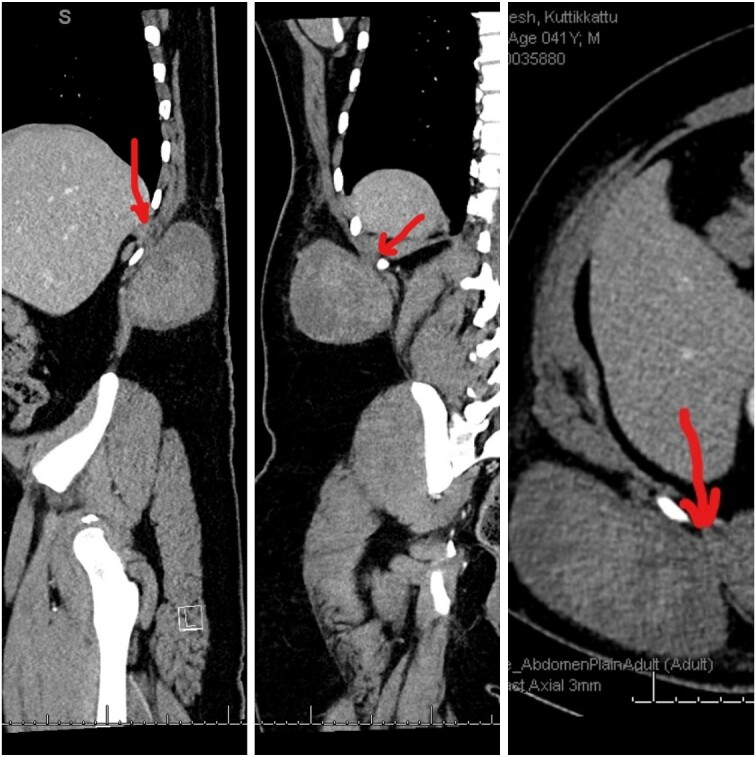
CT images showing the heterogenous lesion in the right flank with the loss of fat plane between the lesion and the underlying muscle at one place.
